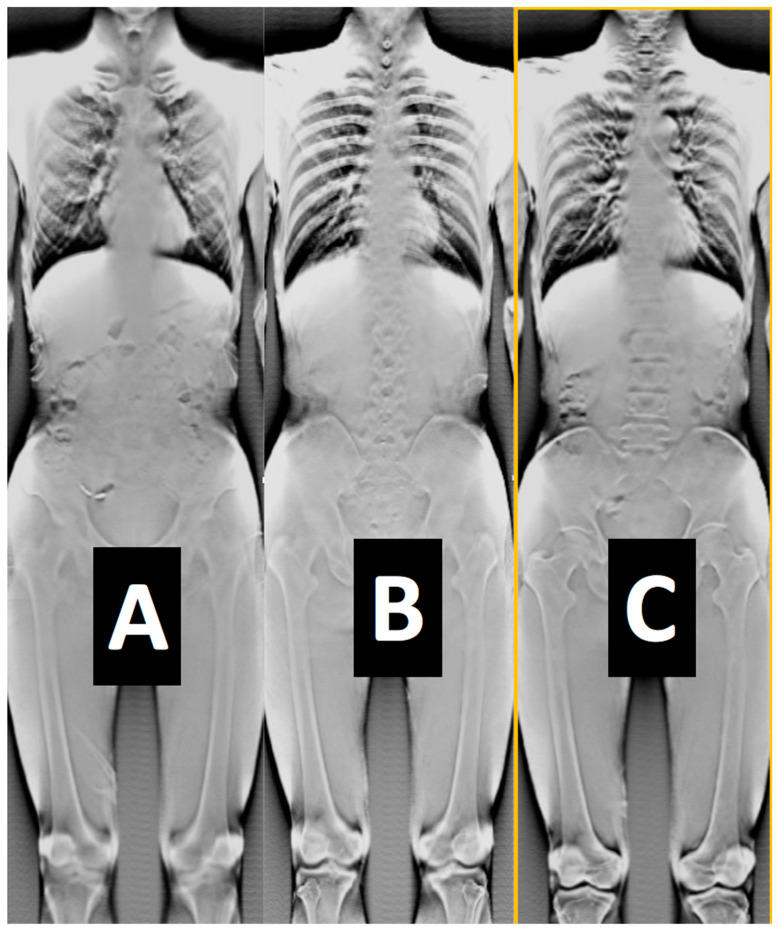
Tomosynthesis (40 μm) images using a dynamically adjusted focal spot (ranging from 0.1 to 0.02 mm) and the geometry for the reconstruction described in Figure 2 shown here as sequences of the whole body of a human standing for an otherwise narrow field of view (*z*-axis). Images (A–C) show three sequences of a full-body tomosynthesis scan with similar settings as the CT (Figure 6 top left) but with different reconstruction geometry, i.e., with a much larger SID of 1100 mm.
